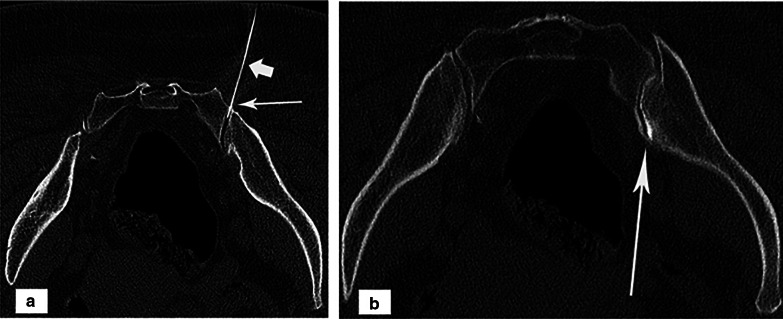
A 50-year-old lady with sacroiliitis referred for SIJ injection. a A non-enhanced CT (bone window) lower cut on SIJ shows the synovial part of the joint (long arrow) where the needle (short arrow) is inserted. b A contrast-enhanced CT (bone window) upper cut reveals contrast (long arrow) within the anterior synovial part of the joint. No contrast in the posterior fibrous part of the joint
